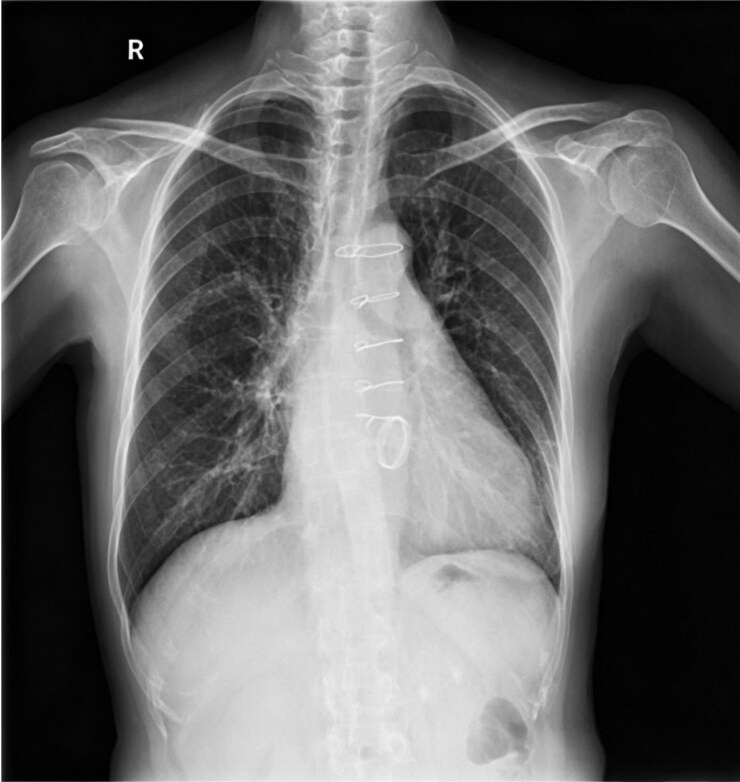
Chest radiographs at 3 months postoperatively.
